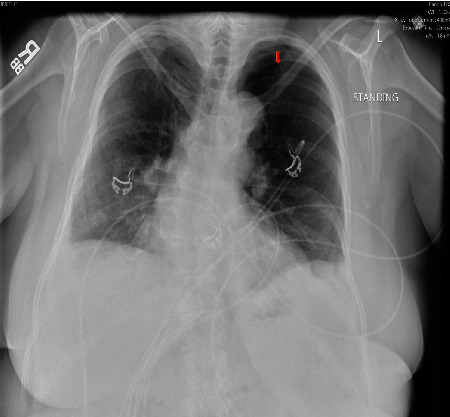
Chest X-ray showing large left-sided pneumothorax (depicted by red arrow).
